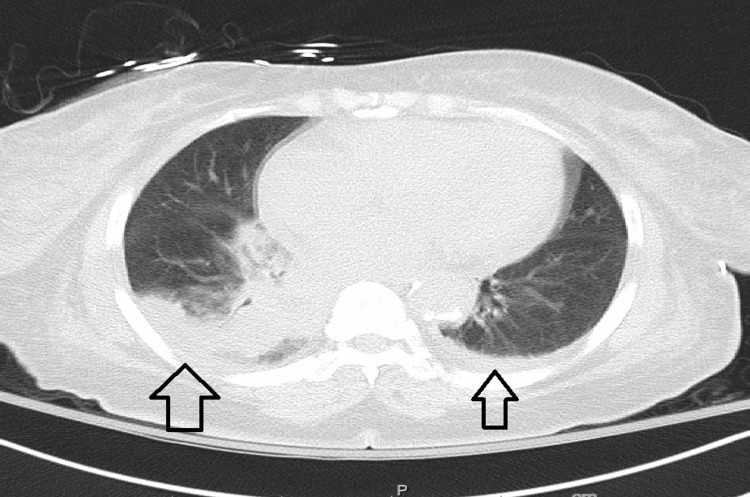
Computed tomography of chest showing bilateral posters-basal infiltration with effusion (arrows).
